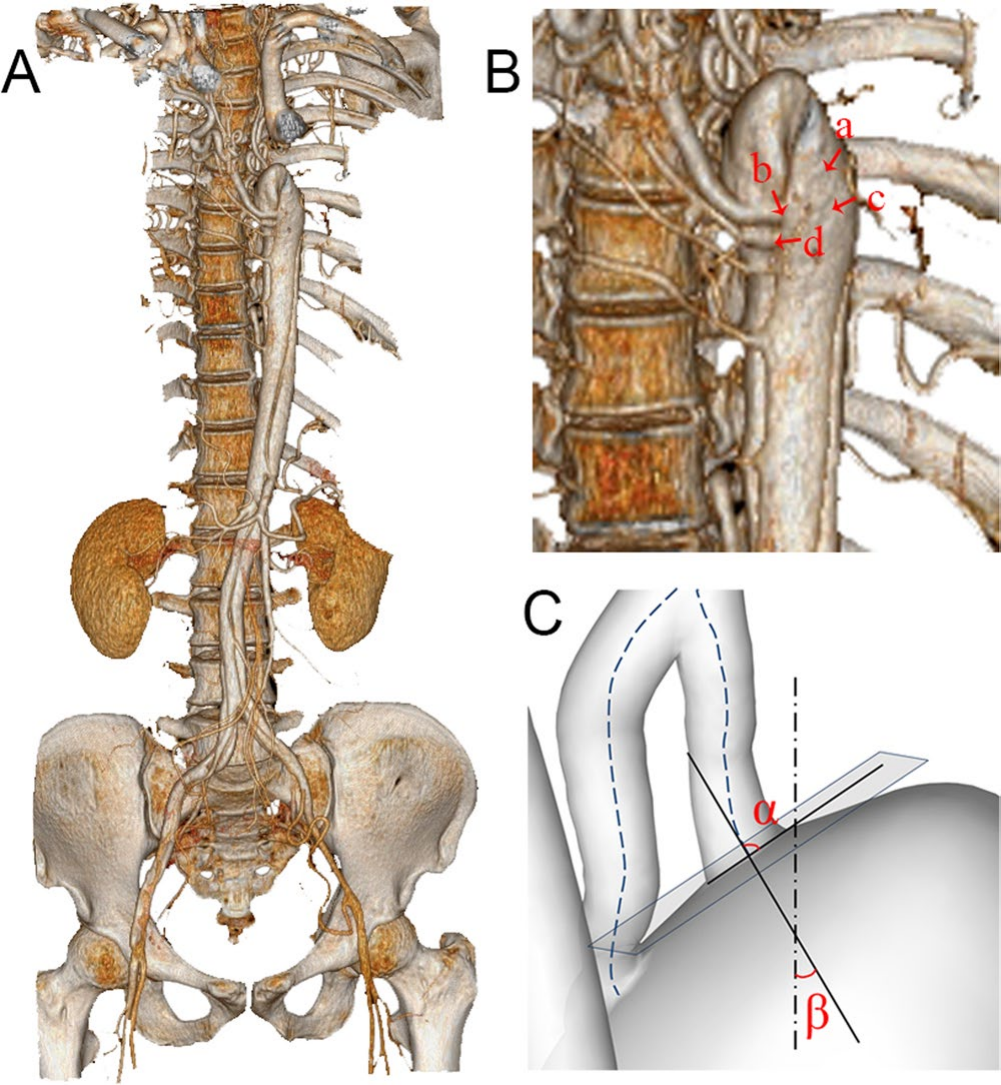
3D reconstruction of IAA model. (A) The reconstructed geometry with dissection using CT images; (B) Four tears a, b, c and d are marked with red arrows; (C) Angles α (the angle between the axis line and the tangent plane) and β (the angle between the axis line and the vertical axis) of CA_1_ are demonstrated. (CT = computed tomography; CA = collateral artery).
